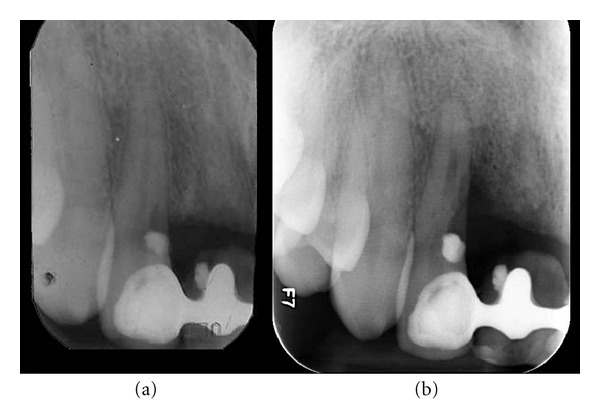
Patient 1: radiographs of the right maxillary central incisor region. (a) 2000. The radiograph demonstrates a filling in the mesial aspect of the lateral incisor and resorption below this filling. The alveolar bone level has not changed remarkably since the situation demonstrated in Figure 4(d). (b) 2012. The radiograph demonstrates severe bone loss of the alveolar bone mesially to the lateral incisor root. The resorption process below the filling has progressed.
